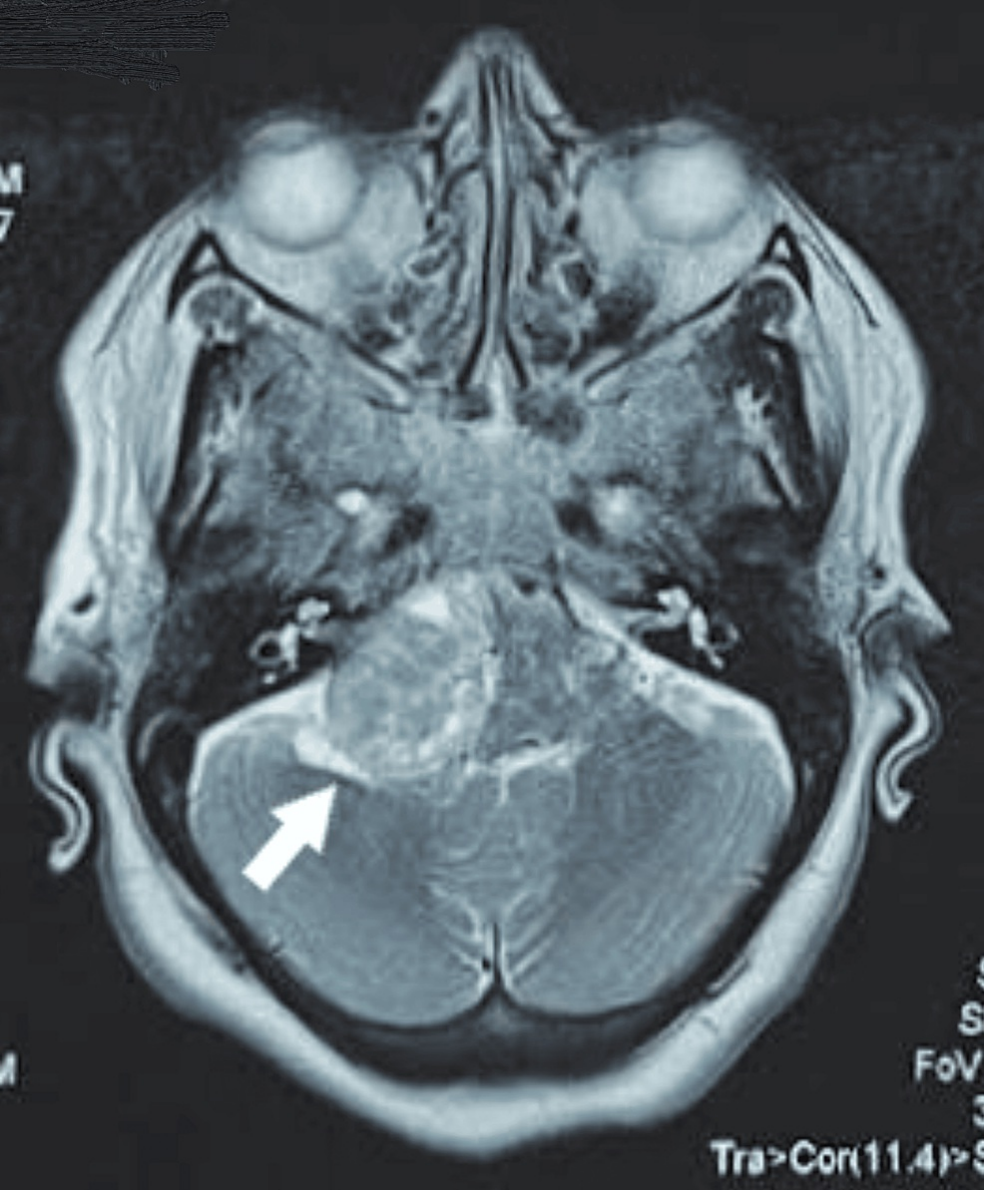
MRI Brain (transverse section) at the level of internal acoustic meatus showing typical "ice-cream cone" appearance of the tumor, compressing structures at the cerebellopontine angle .
